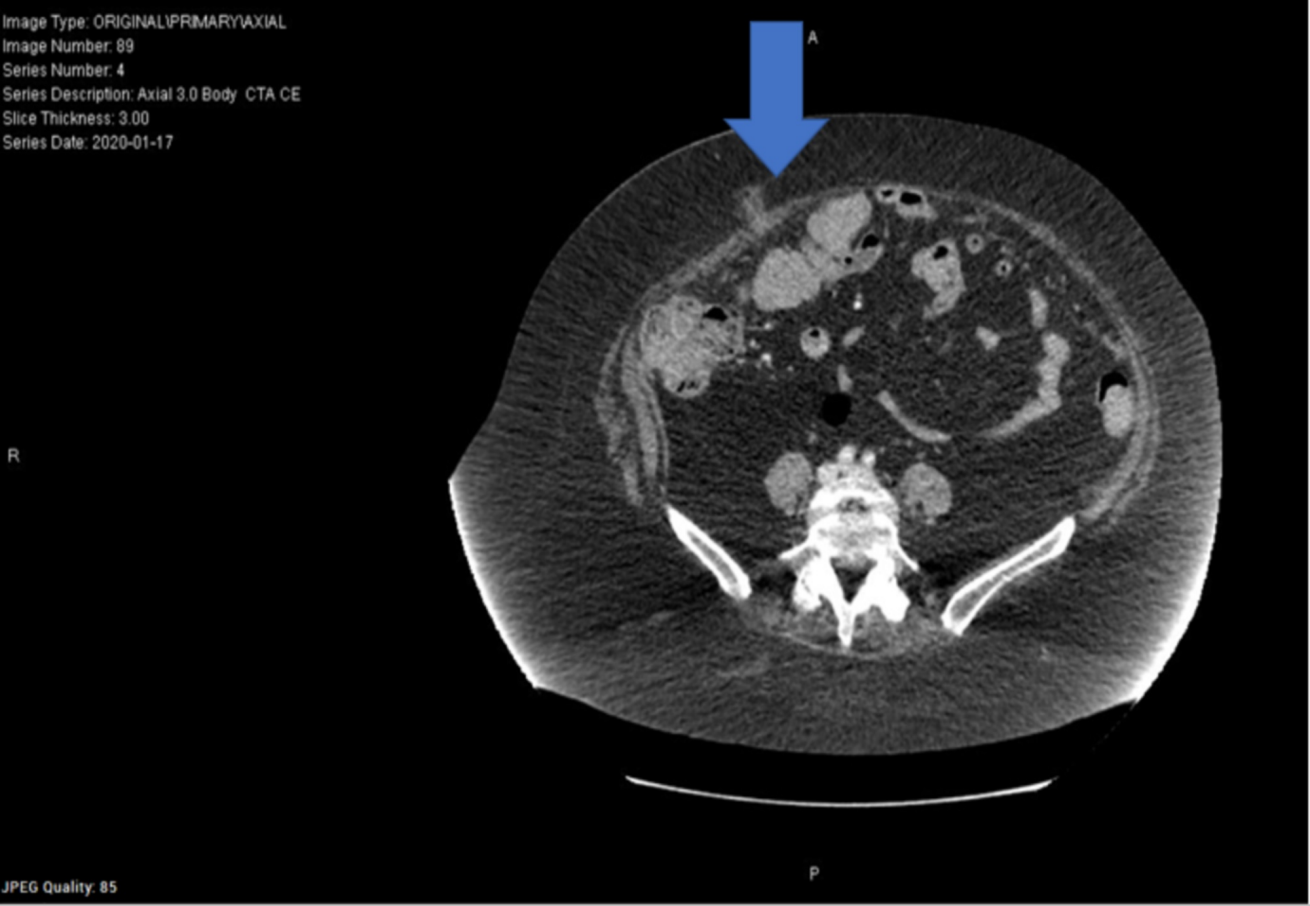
Repeat CT abdomen - Nodularity in the omentum particularly in the lower abdomen concerning for omental metastasis. Structures anterior to the falciform ligament within the peritoneal fat. These findings are increased/new from prior imaging. There is a concern these may represent omental metastasis. CT: computed tomography
